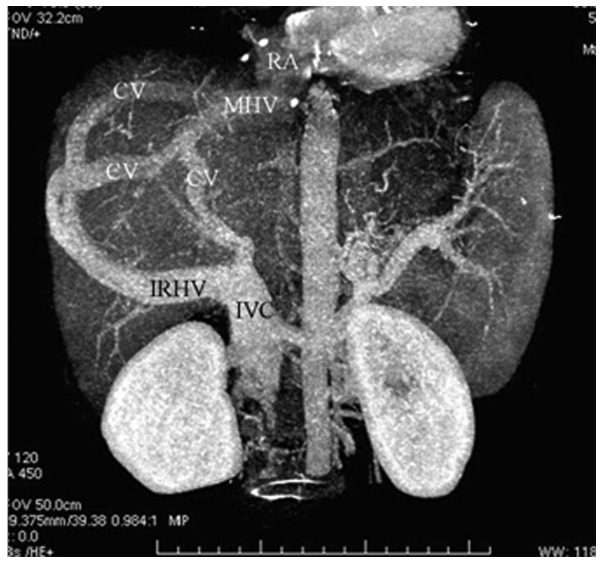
IVC-HV/accessory HV-HV-right atrium collaterals. Computed tomography angiography shows segmental occlusion of the IVC. Blood flow within the IVC reverses to the IRHV and then continues through the CVs to the MHV and then to the RA. IVC, inferior vena cava; HV, heptatic vein; IRHV, inferior right HV; CV, communicating vein; MHV, middle HV; RA, right atrium.
